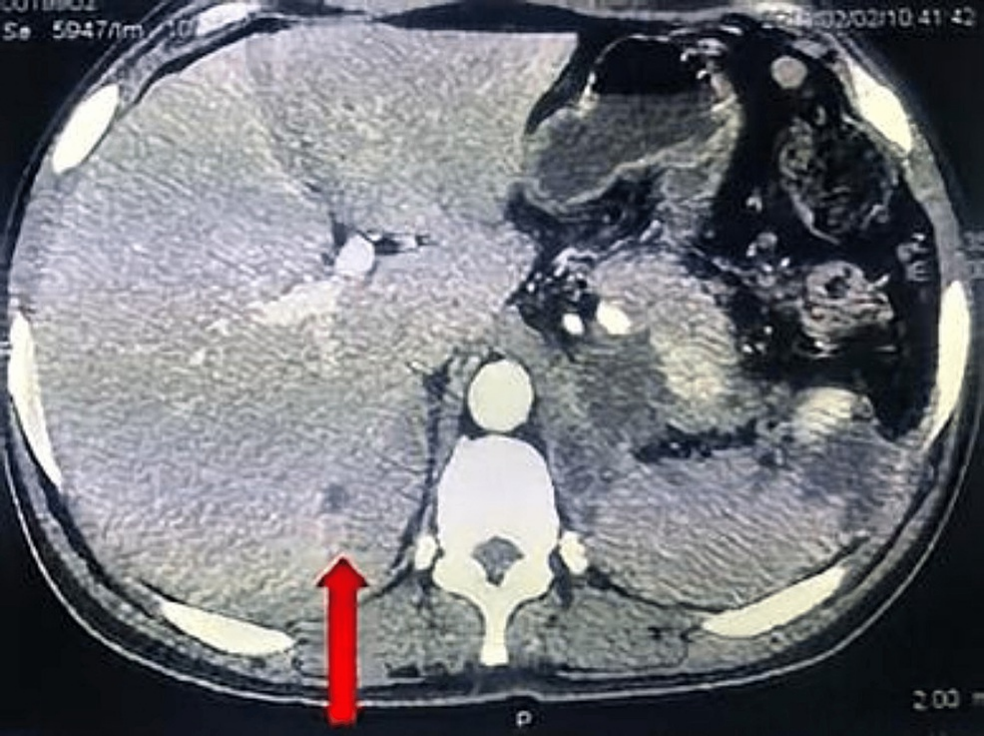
A contrast CT abdomen transverse view showing new liver metastasis (arrow).
